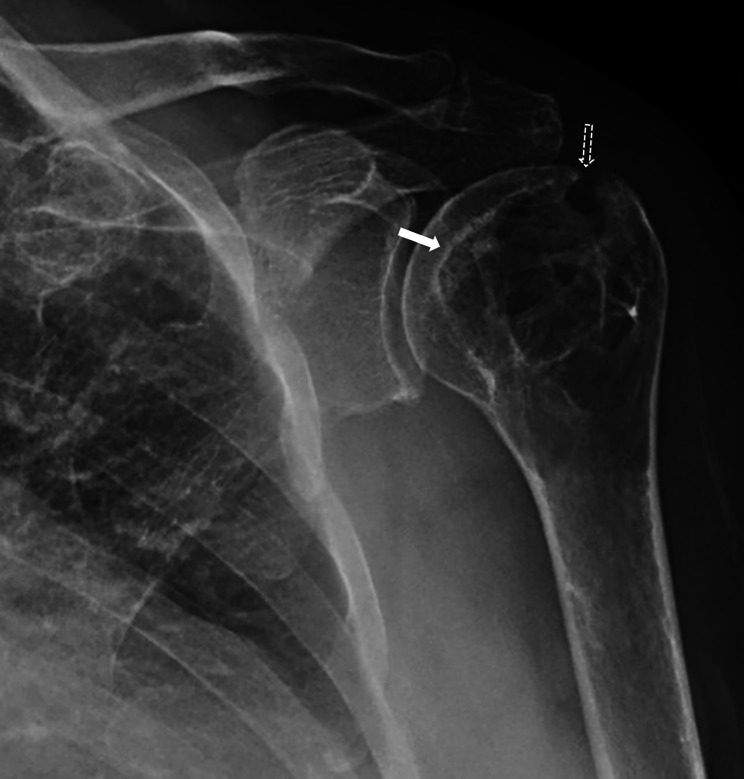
X-ray of the left shoulder in the RA patient. Anteroposterior radiograph shows expansive intramedullary osteolytic lesion, at the metaepiphysis region of the humerus, with well-defined contours and sclerotic border (white arrow), presenting internal septations. The image also shows an erosive lesion on the superolateral region of the humeral head, with slightly cortical thinning alongside (dot arrow)
